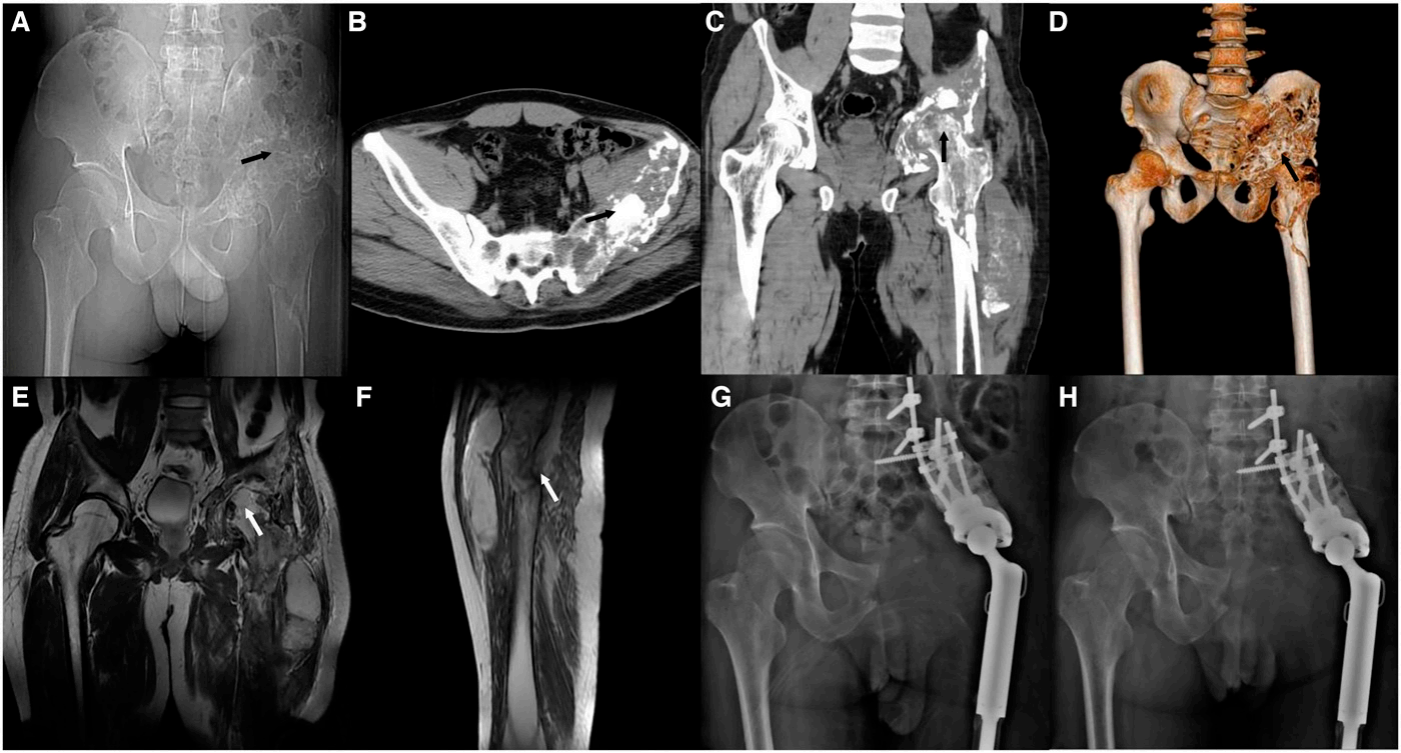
A 30-year-old man presented with progressive, painful limitation of the left hip for 6 months. (A) Preoperative anteroposterior X-ray of the pelvis showed osteolytic bone destruction in the left ilium, periacetabulum, pubic bone, ischium, and proximal femur, with interruption of cortical continuity in the left proximal femur (black arrow). (B–D) Preoperative computed tomographic scans and three-dimensional reconstruction showed multiple areas of low-density bone destruction in regions I, II, and III of the left pelvis and proximal left femur (black arrows). (E, F) Preoperative magnetic resonance images showed multiple, round, long T2 signals of different sizes in the left ilium with surrounding soft tissues, left pelvic wall, and left buttock muscle (white arrows). (G, H) Postoperative and postoperative month 3 anteroposterior X-rays of the pelvis showed good alignment of the femoral prosthesis and pelvic internal fixation.
